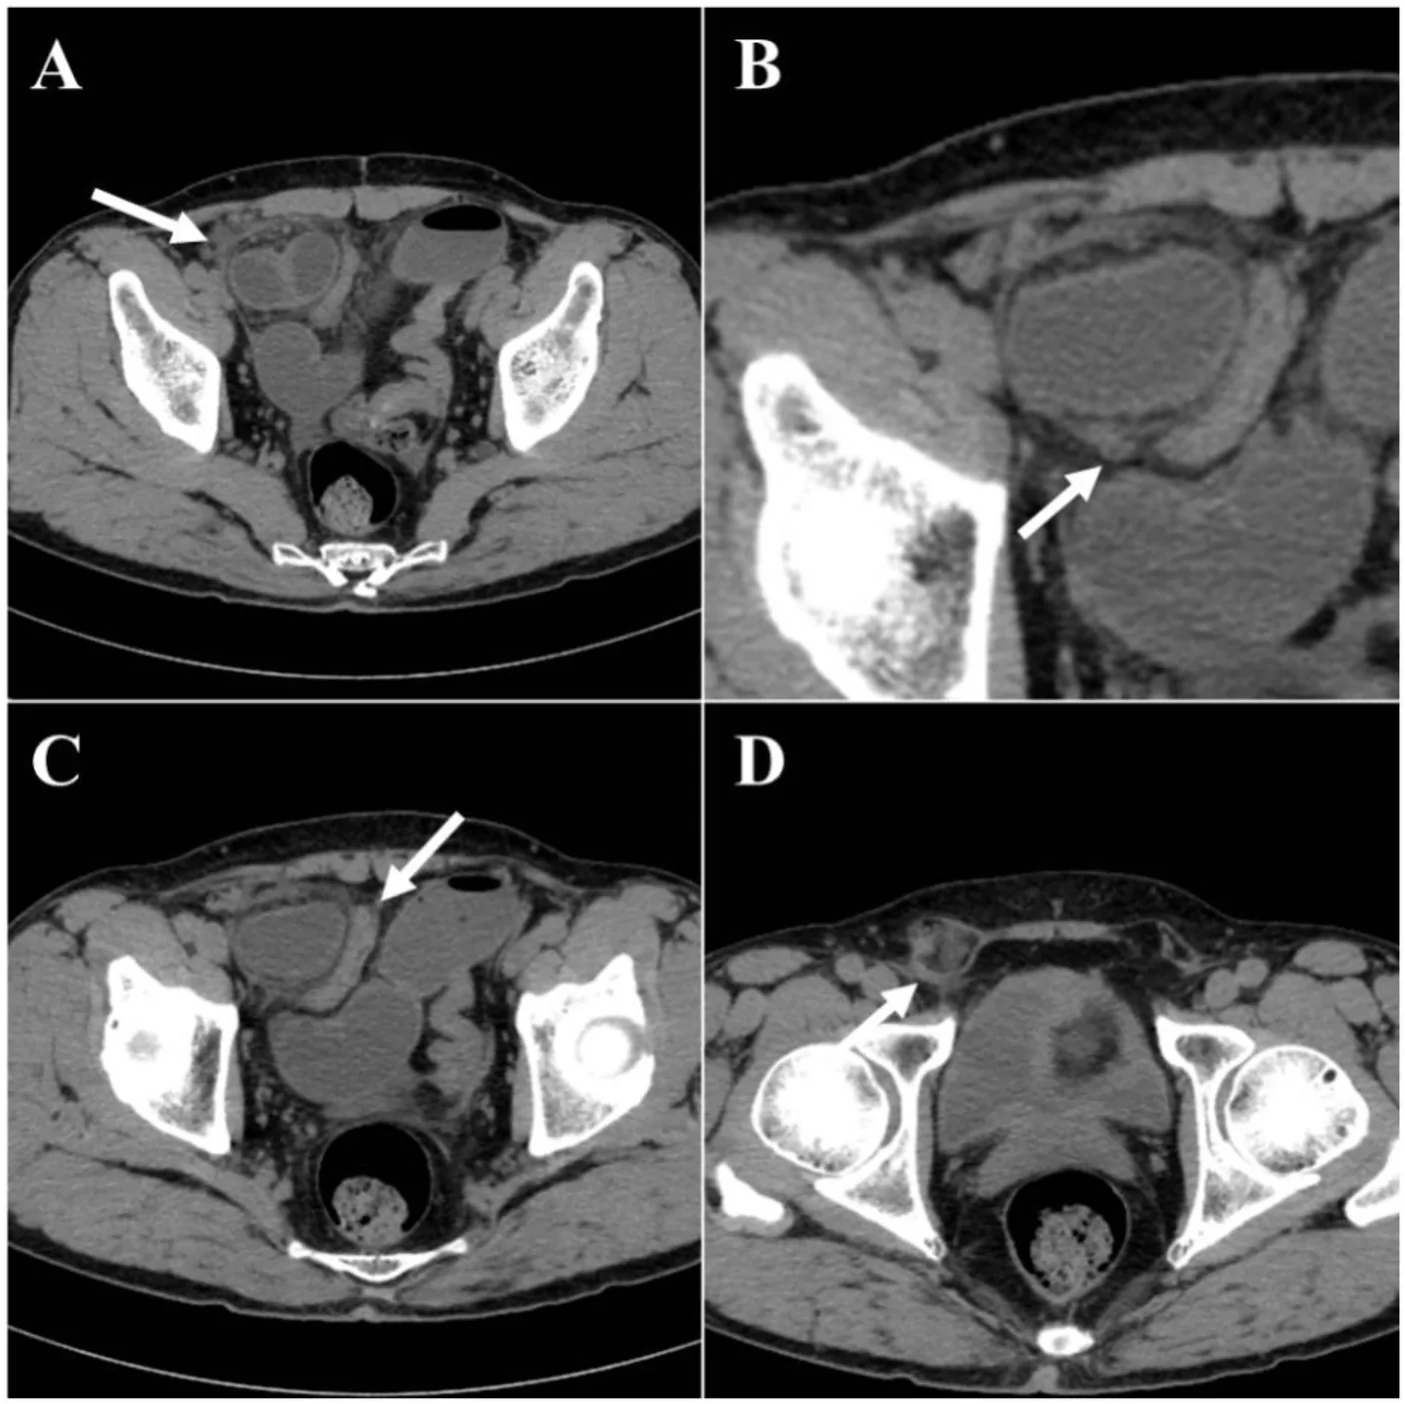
Initial abdominal CT scan images after the patient's onset. (A) The arrow indicates a spherical intestinal loop adjacent to the right groin. (B) The arrow indicates a circular cord-like structure at the site of obstruction. (C) The arrow indicates the bladder with a beak-like deformation along the closed intestinal loop. (D) The arrow indicates that the soft tissue shadow in the right groin area is more prominent than that on the left side.
